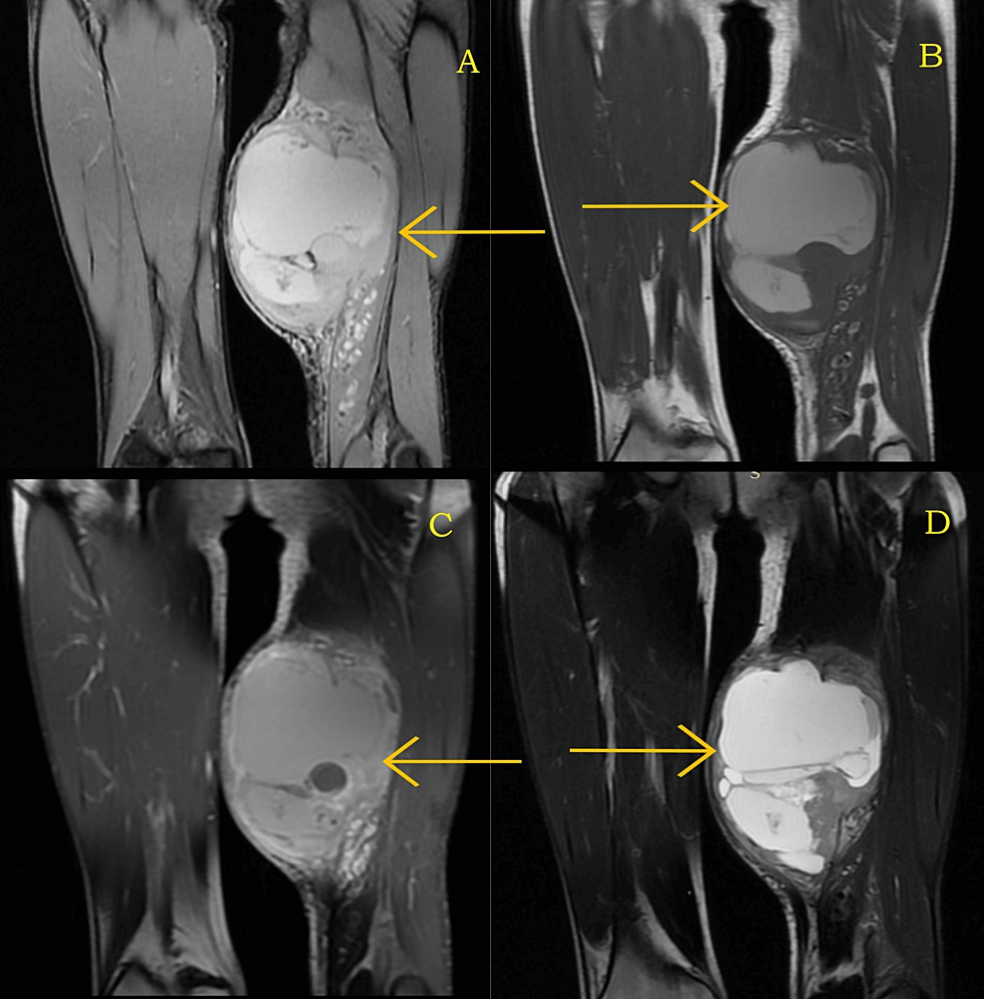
(A-D) Magnetic resonance images of the tumor in the left thigh
